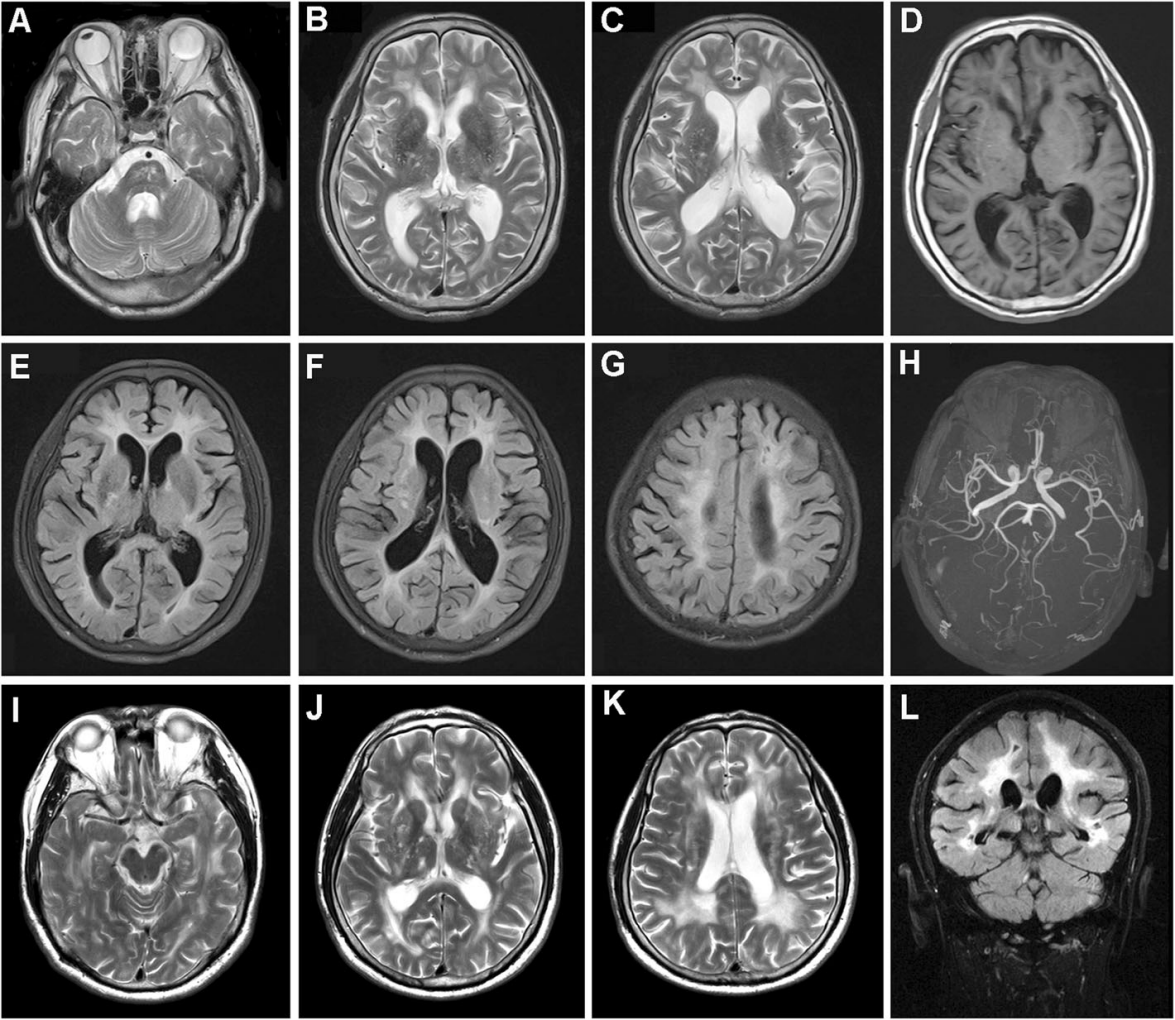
Brain MRI of the patient. Brain MRI of the patient 1 (a-h) and patient 2 (i-l) in the family with CADASIL showed diffused white matter hyperintensities as well as lacunar infarcts on T1-weighted, T2-weighted and FLAIR imaging
